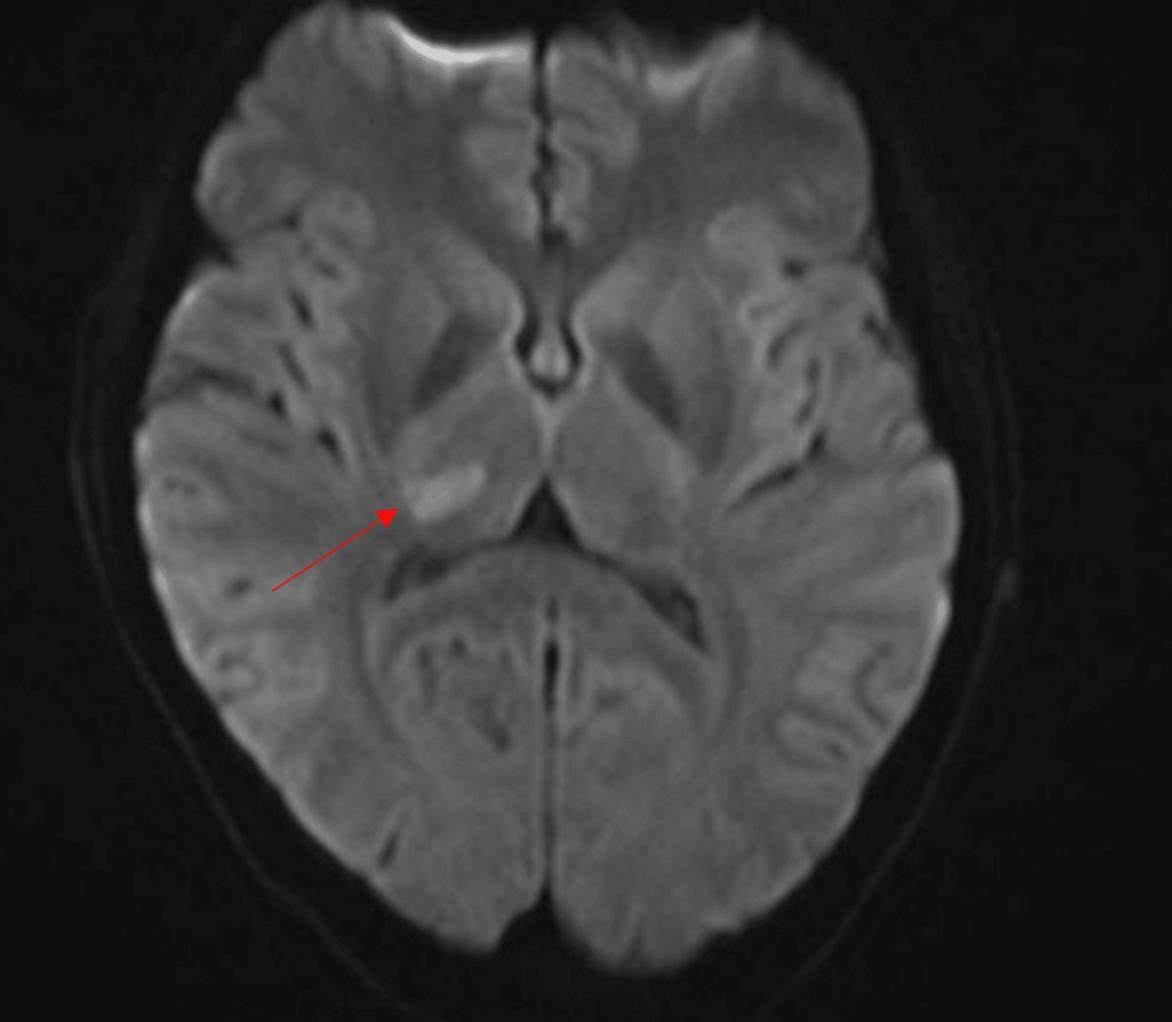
MRI brain shows right thalamic infarct
